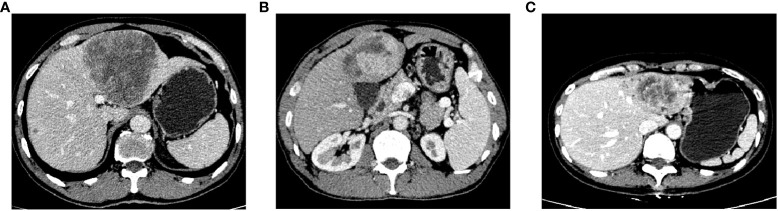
Enhanced CT for PTC (A), IRE (B), and both PTC and IRE (C), CT, computed tomography; PTC, peritumor capsule; IRE, rim-like arterial phase enhancement.
